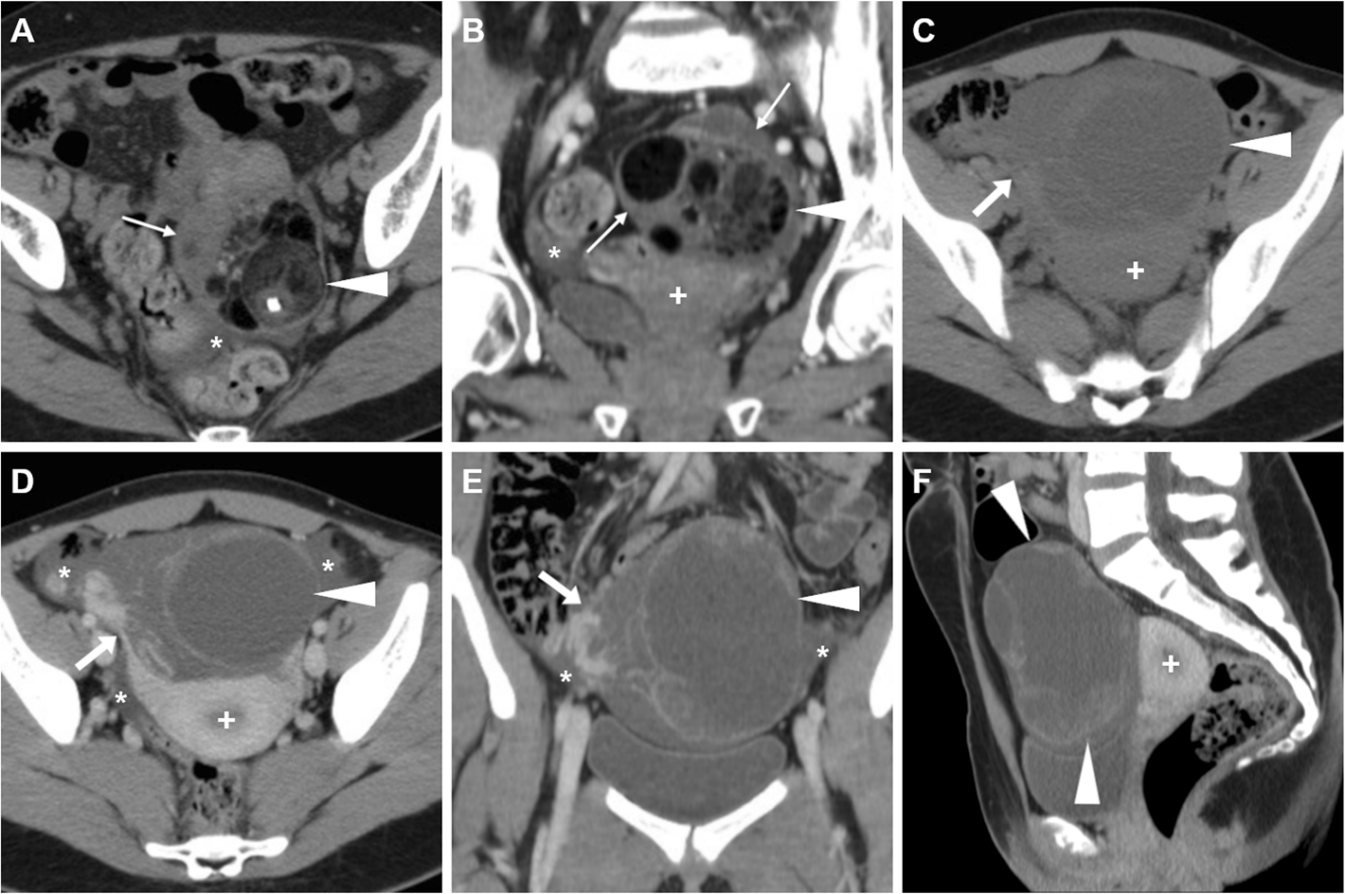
Two surgically proven cases of adnexal torsion. a, b Axial (a) and coronal (b) CT images show enlarged left ovary (arrowheads) with thickened oedematous periphery (thin arrows), containing a mixed-attenuation roundish mass with fat-attenuation foci and a calcification, corresponding to a mature cystic teratoma. Note the ipsilateral attraction of uterus (+), minimal fluid in the peritoneal cul-de sac (asterisk in a). c–f Unenhanced (a) and post-contrast (d–f) CT images show large midline pelvic mass (arrowheads) consistent with malignant teratoma with poor, irregular enhancement that displaces the uterus and bladder. Note the peritoneal effusion (asterisk) and congested ovarian vessels on the right side
